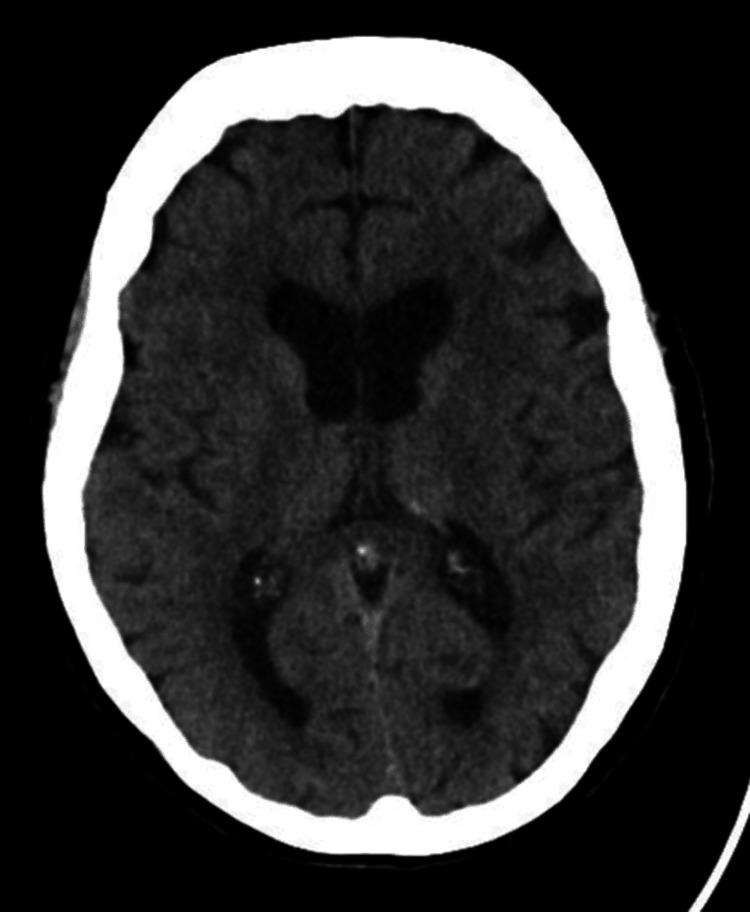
CT head showing no acute parenchymal infarction
